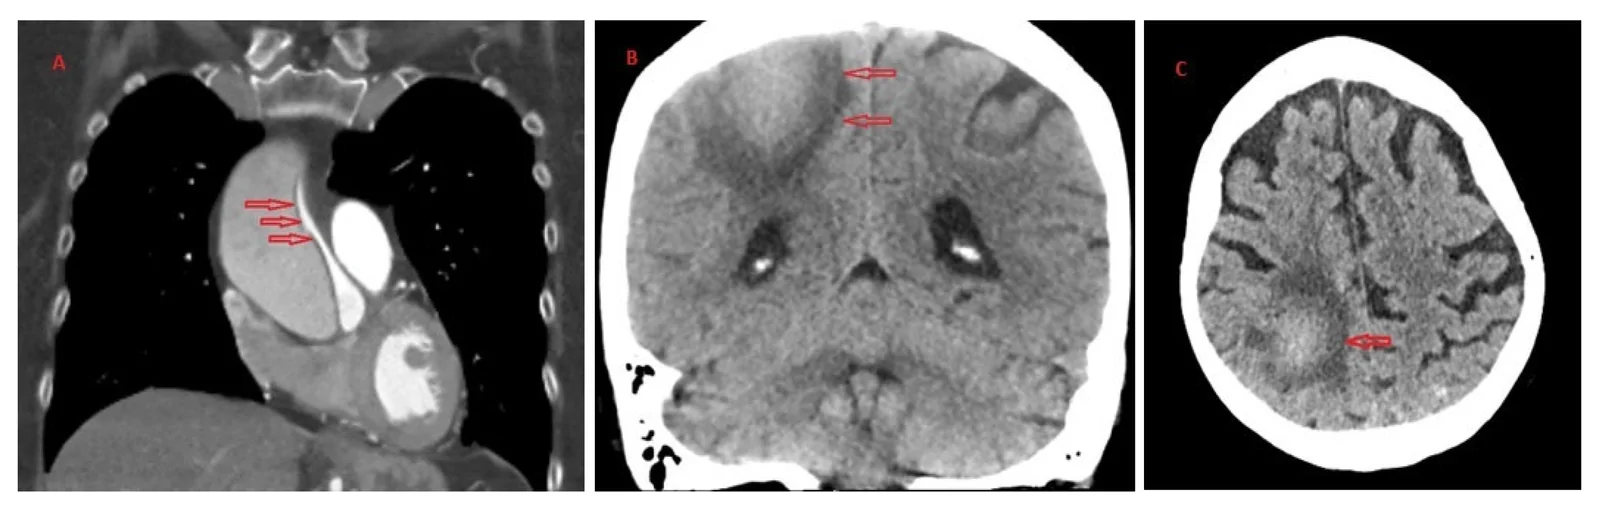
CT imaging four weeks after admission showing progressive collapse of the true lumen (A) and beginning resorption of the intracerebral haematoma (B,C).
